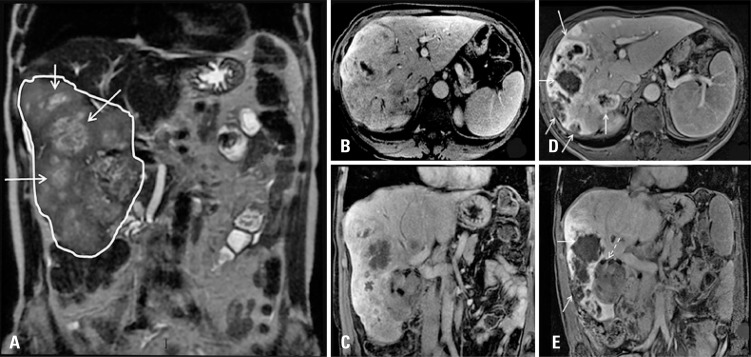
(A) Magnetic resonance imaging of upper abdomen in T2, with no fat saturation, in coronal section, showing bulky hepatic tumor (metastases) located preferably in the right hepatic lobe (white line). Diffuse heterogeneity of coalescent lesions, with high signal central areas (white arrows). (B-E) Magnetic resonance imaging of upper abdomen in T1, with fat saturation, after contrast, in the axial and coronal sections: in B and C, before SIRT, note the bulky hepatic tumor (metastases) located, preferably in the right lobe. In D and E, 2 months after SIRT, there are extensive necrotic areas of infiltrating lesions in the right hepatic lobe (white arrows). Complete necrosis of the tumor lesion in the gallbladder bed stand out (dotted white arrow)
